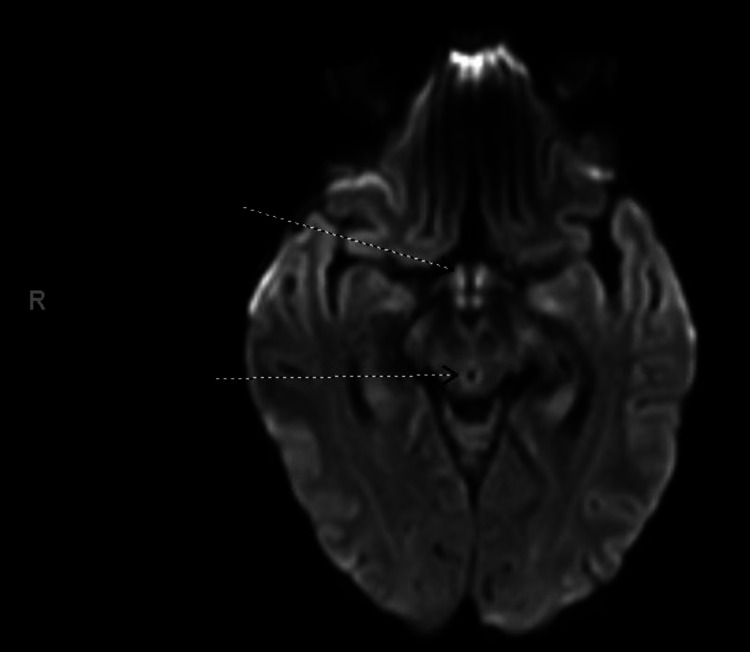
T2-FLAIR hyperintensities in the periaqueductal gray, medial thalamus, and mamillary bodies that were consistent with non-alcoholic Wernicke encephalopathy. FLAIR: fluid-attenuated inversion recovery
